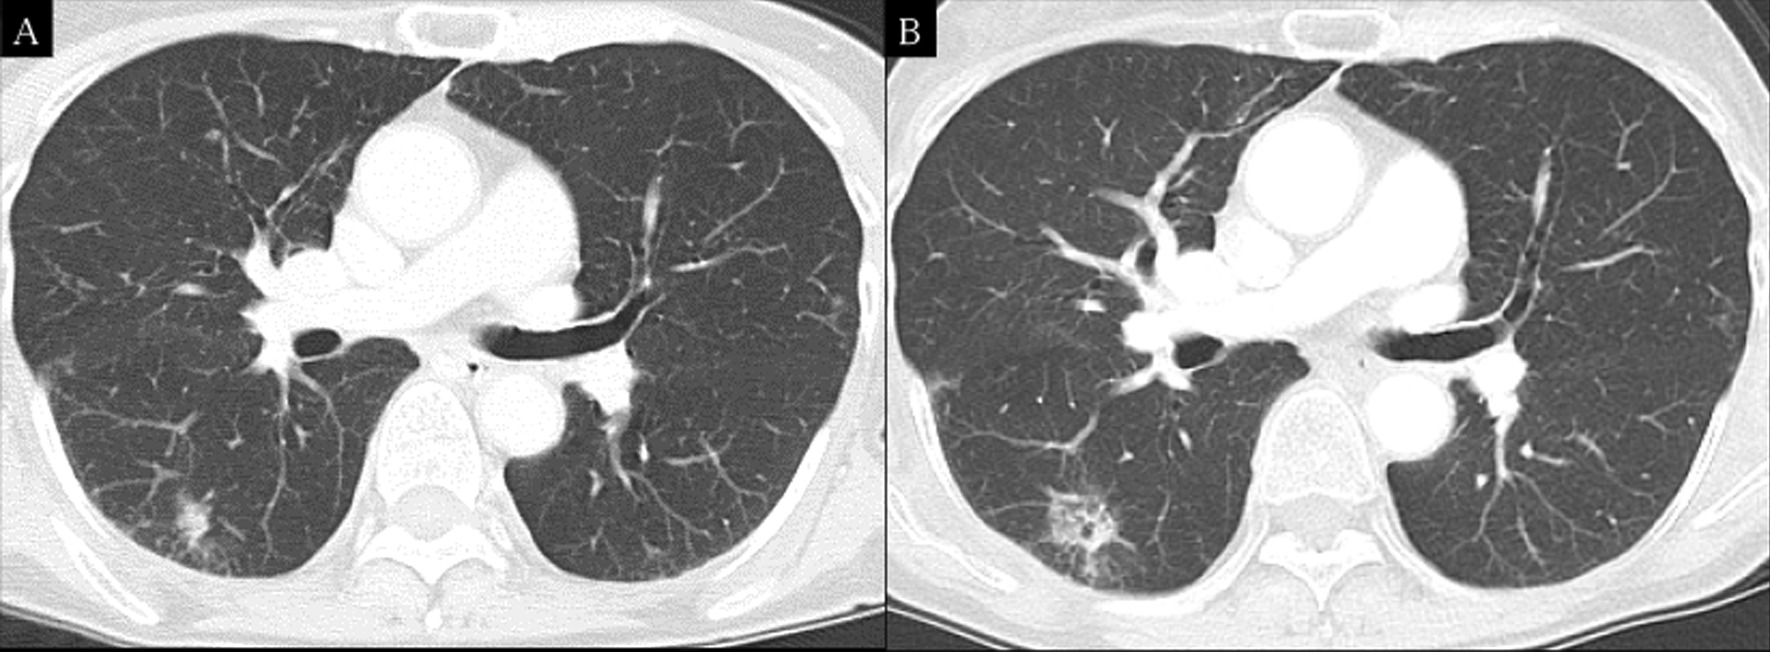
Chest computed tomography. A Semi-solid ground-glass attenuation (GGA) in the right lower lobe, B a slow-growing GGA in 1.5 years
